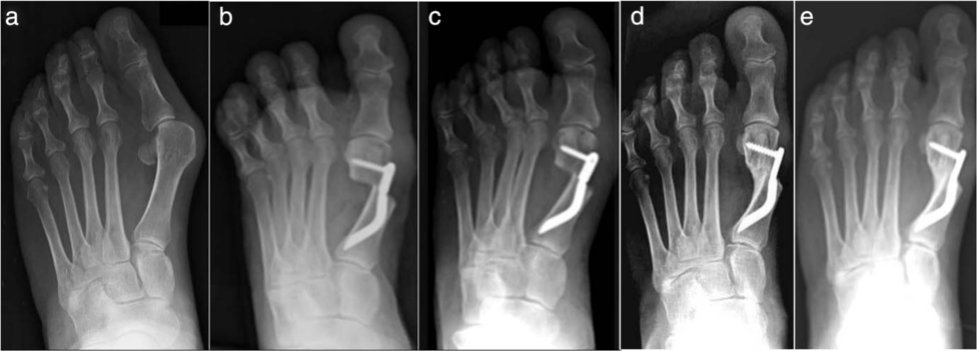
Case 10. A 59-year-old woman with severe HV; antero-posterior radiographic images at: a Preoperative period. b 1-month follow-up. c 3-month follow-up. d 6-month follow-up. e 48-month follow-up showing the maintained correction of the deformity
